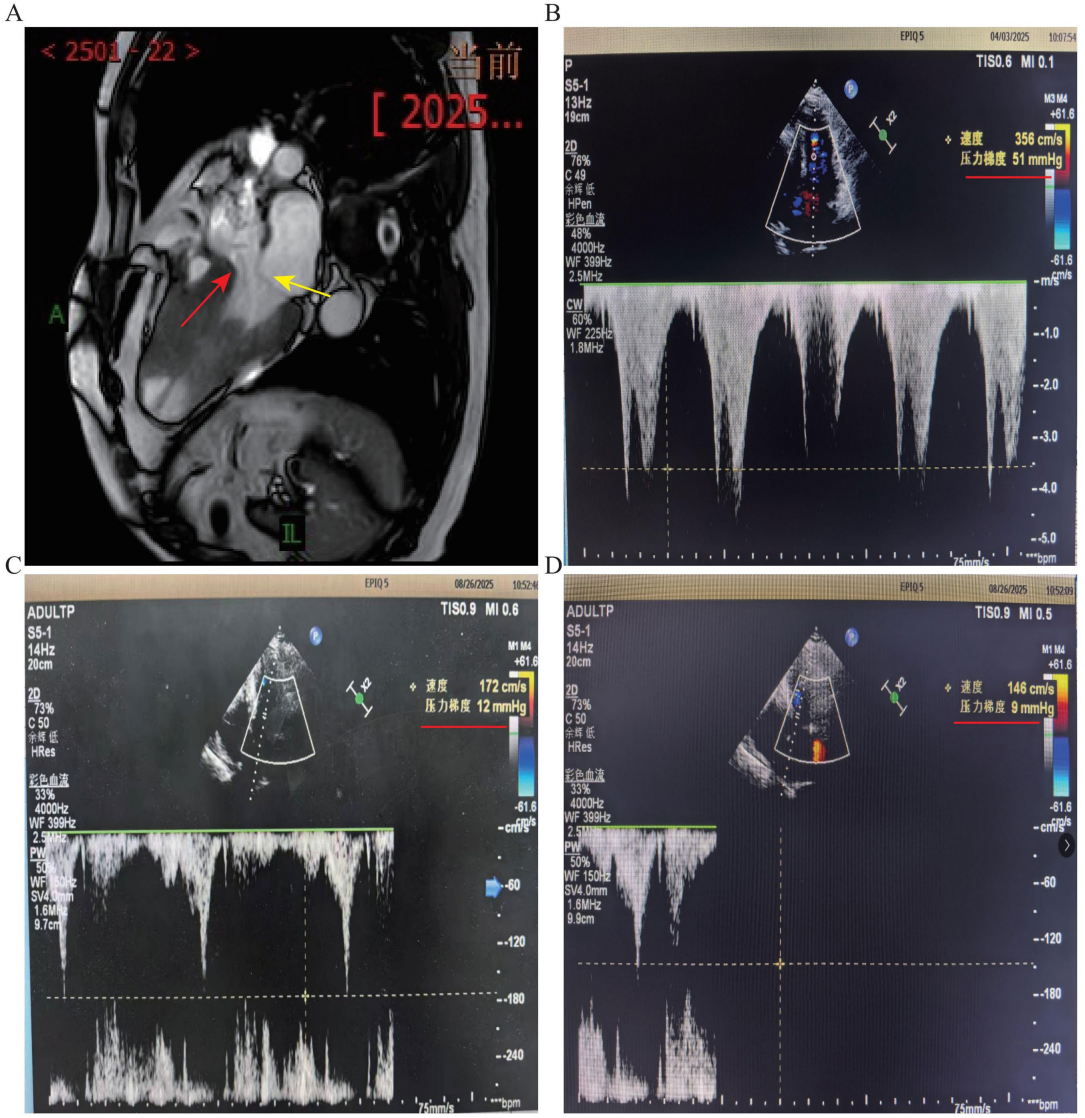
Cardiac magnetic resonance imaging and transthoracic echocardiography after PCI intervention in the RCA and LCX. (A) Post-intervention cardiac magnetic resonance imaging. Red and yellow arrows indicated the left ventricular hypertrophy and left ventricular outflow tract obstruction, respectively. (B) Transthoracic echocardiography after PCI in the RCA and LCX. (C) Resting transthoracic echocardiography after 4 months of mavacamten treatment. (D) Transthoracic echocardiography during the Valsalva maneuver.
